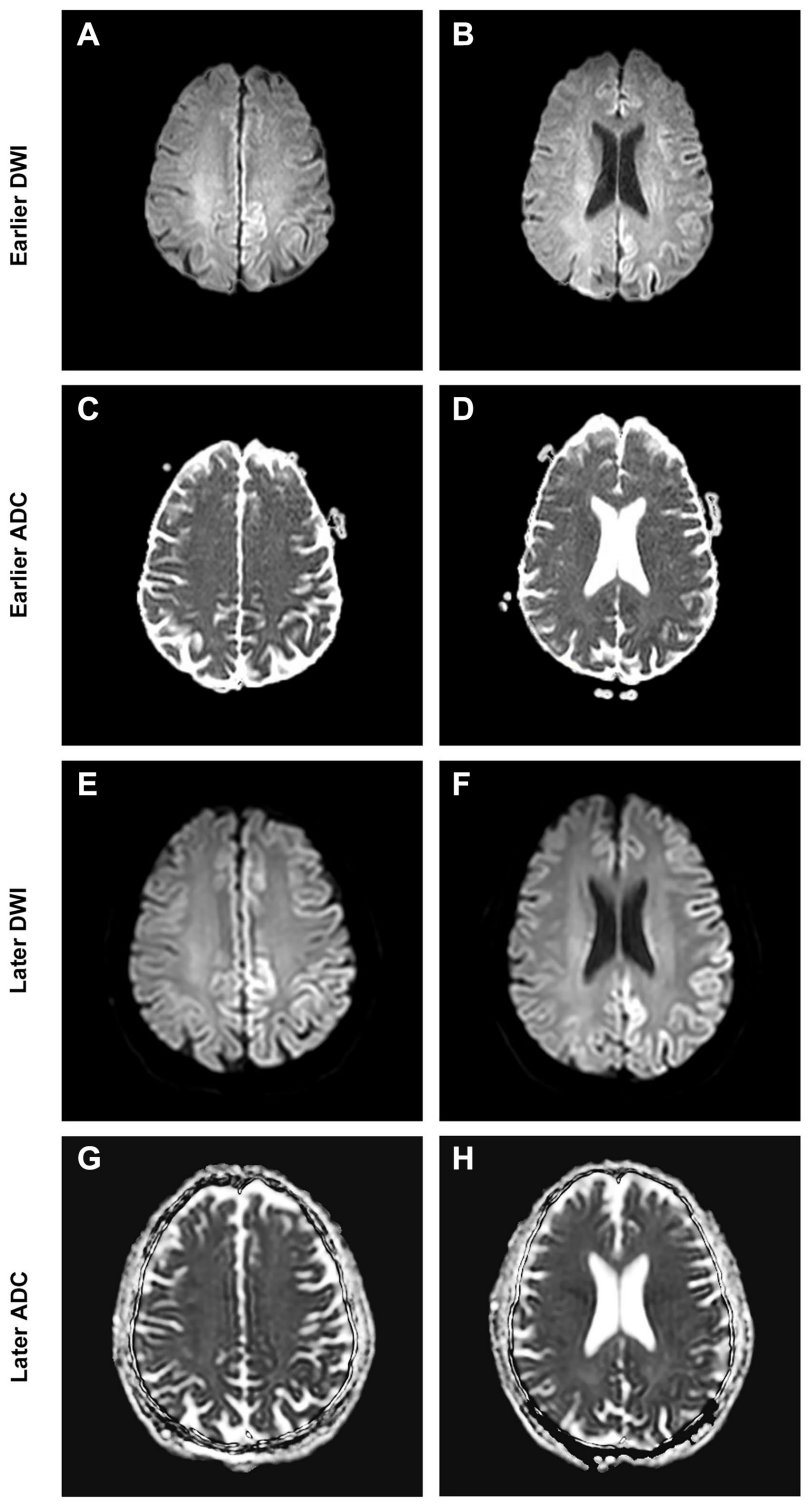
Brain MRI at presentation shows cortical DWI hyperintensities “cortical ribboning” (A,B) with ADC hypointensity (C,D) over the corresponding cortex. Brain MRI at follow-up shows spreading of the cortical restriction in DWI (E,F) and ADC (G,H) currently involving the left frontal, parietal, temporal, and occipital lobes.
